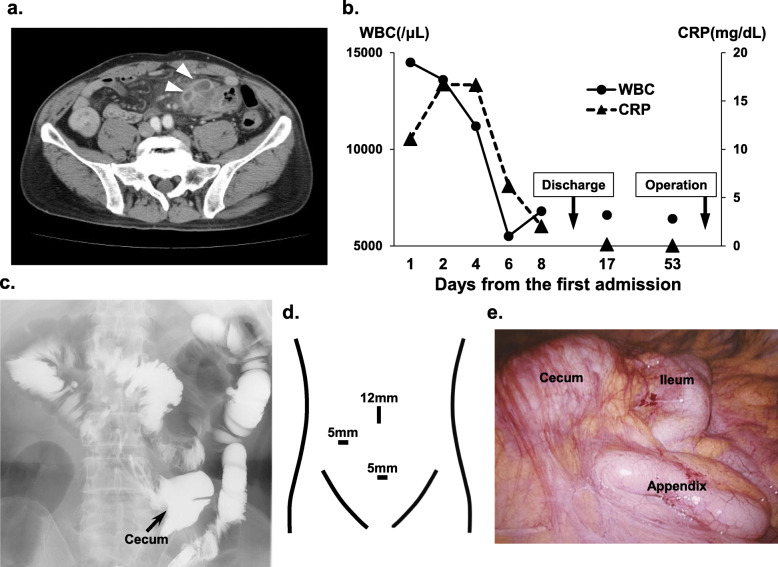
a CT findings: the cecum was located in the left lower side of the abdomen. The swollen appendix was surrounded by intra-abdominal abscesses (arrow heads). b The clinical course of this patient: he was discharged at day 9 and underwent an interval appendectomy at day 54. c The gastrografin enema findings: the cecum (arrow) located in left-lower side of the abdomen. d The trocar placement of the interval appendectomy: Note the mirror image trocar placement against an ordinal laparoscopic appendectomy. e Intraoperative findings: iliocecal structures were located in the left lower abdomen
